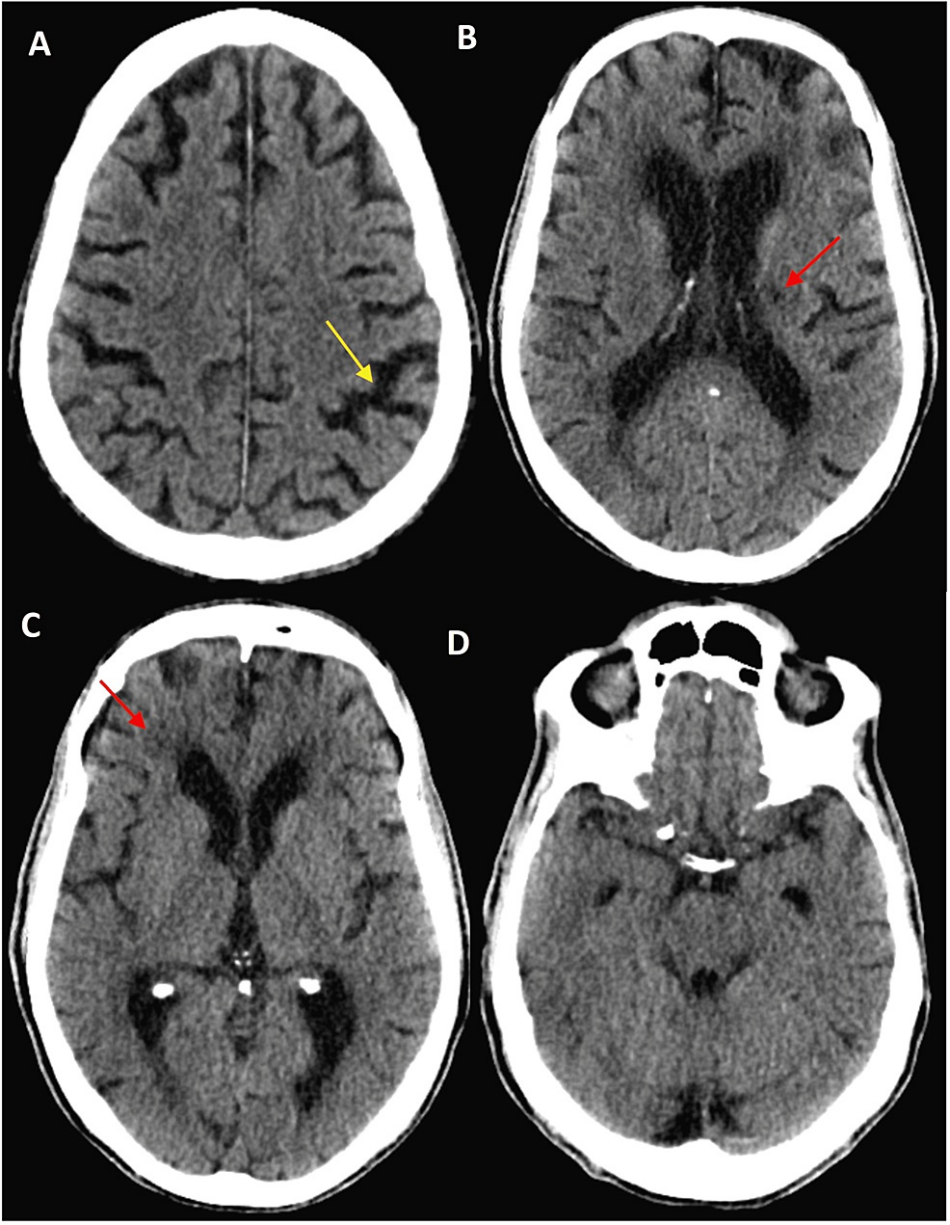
CT head without contrast of the patient. The images show (A) parenchymal volume loss (yellow arrow) and (B and C) chronic white matter microvascular ischemic disease (red arrows); (D) the posterior cranial fossa and anatomy are essentially normal for the patient's age.
